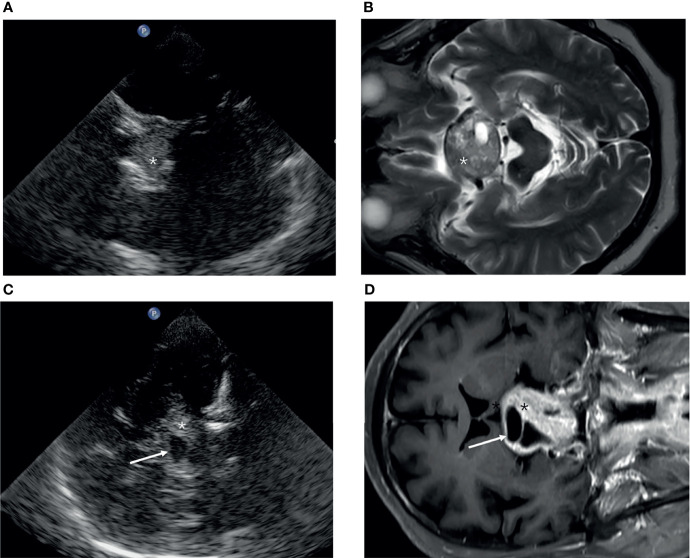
The appearance of a pituitary tumor (asterisk) in transcranial sonography (A, C) and MRI (B, D). (A, B) Axial plane. (C, D) Coronal plane.
